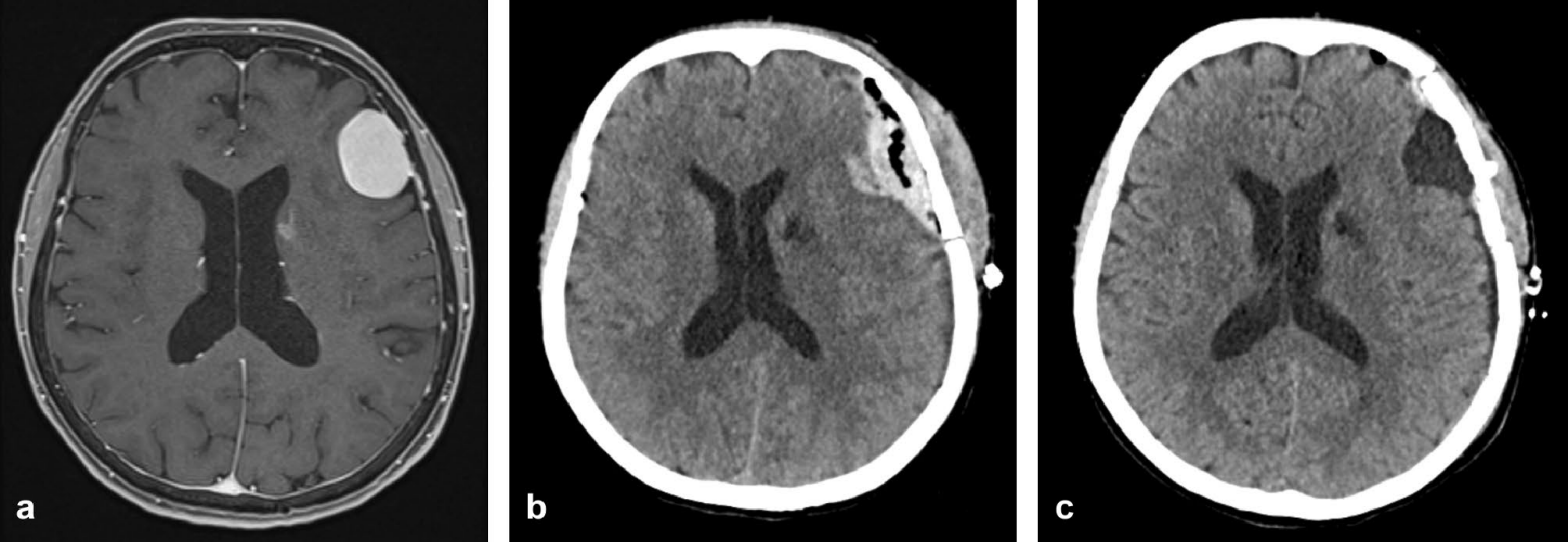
(a) A 71-year old female patient (no. 10) with a history of one (focal) preoperative seizure, who underwent surgery for a left frontal convexity meningioma. (b) On the fourth postoperative day, the patient suffered another focal seizure, yet exhibited no focal or other neurological deficit. The emergency CT scan showed a relevant epidural hematoma, which was surgically removed. (c) Postoperative CT scan after epidural clot removal. The patient’s further clinical course was uneventful.
